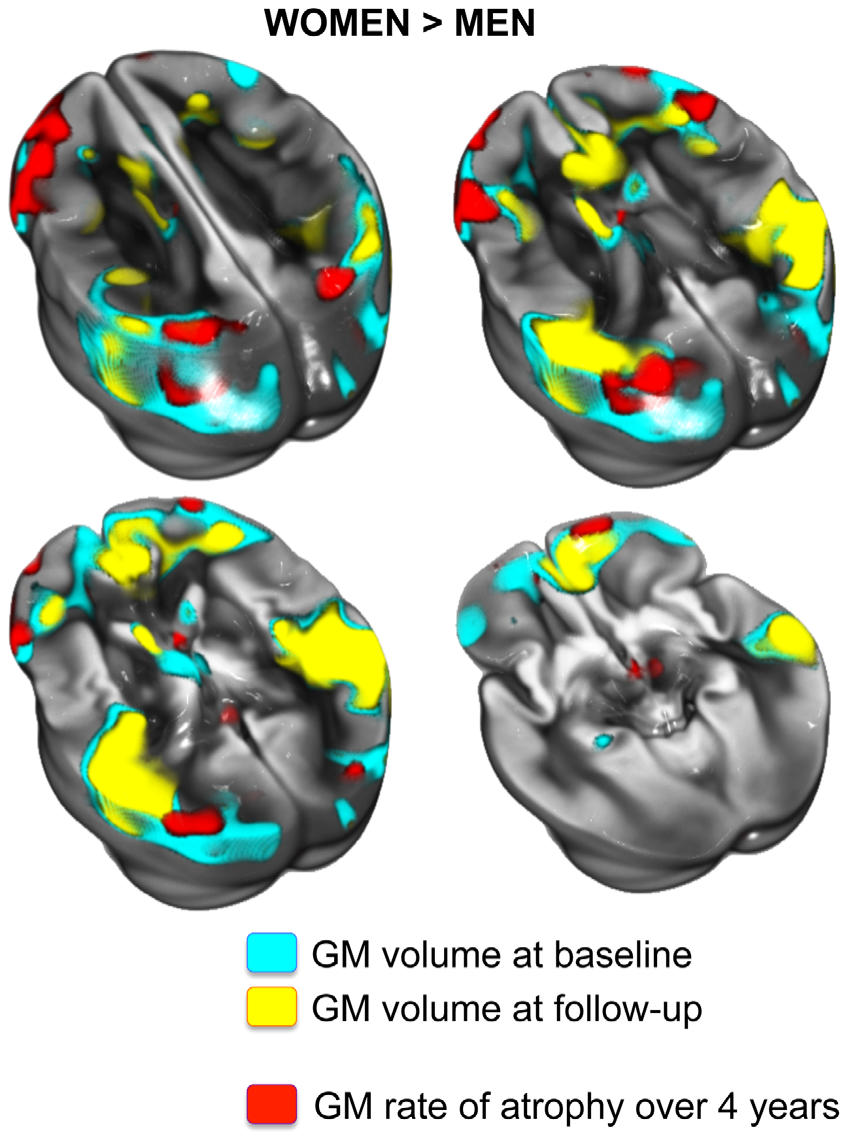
Cortical areas in which women presented a higher GM volume than men at baseline (in blue) and at follow-up (in yellow). Areas where women had a higher rate of atrophy than men are presented in red. These regional sex-related differences are displayed at a significance threshold of *p*<0.05 (FWE corrected) and superimposed onto the mean GM map of the 3C-MRI cohort (*n* = 1,172).
